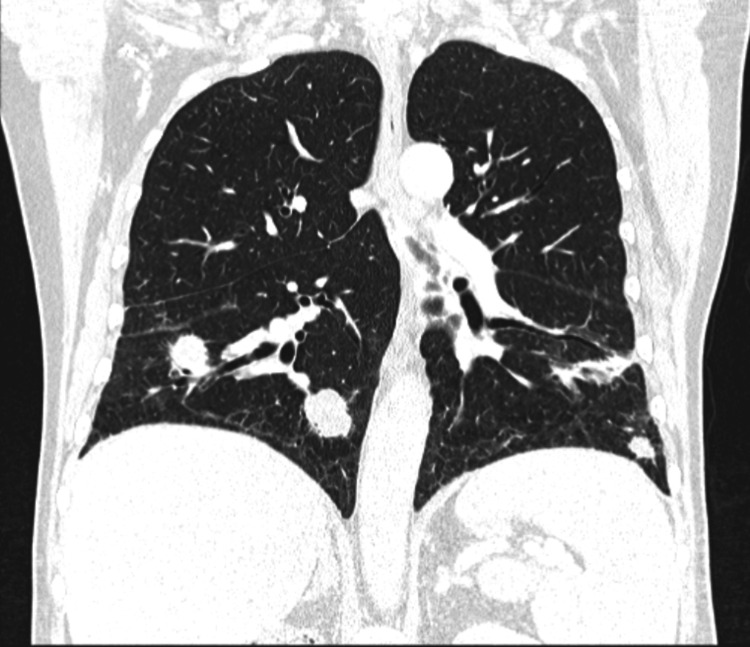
Chest CT (six-month follow-up after immunotherapy initiation) Lung window, coronal view. The largest nodules measured 23 mm (right lower lobe, unchanged), 16 mm (left lower lobe, unchanged), and 14 mm (lingula, previously 16 mm), indicating overall disease stability with reduction in selected lesions. A dimensional reduction of some nodules in the upper lobes and in the apical segment of the left lower lobe was observed. CT: computed tomography
